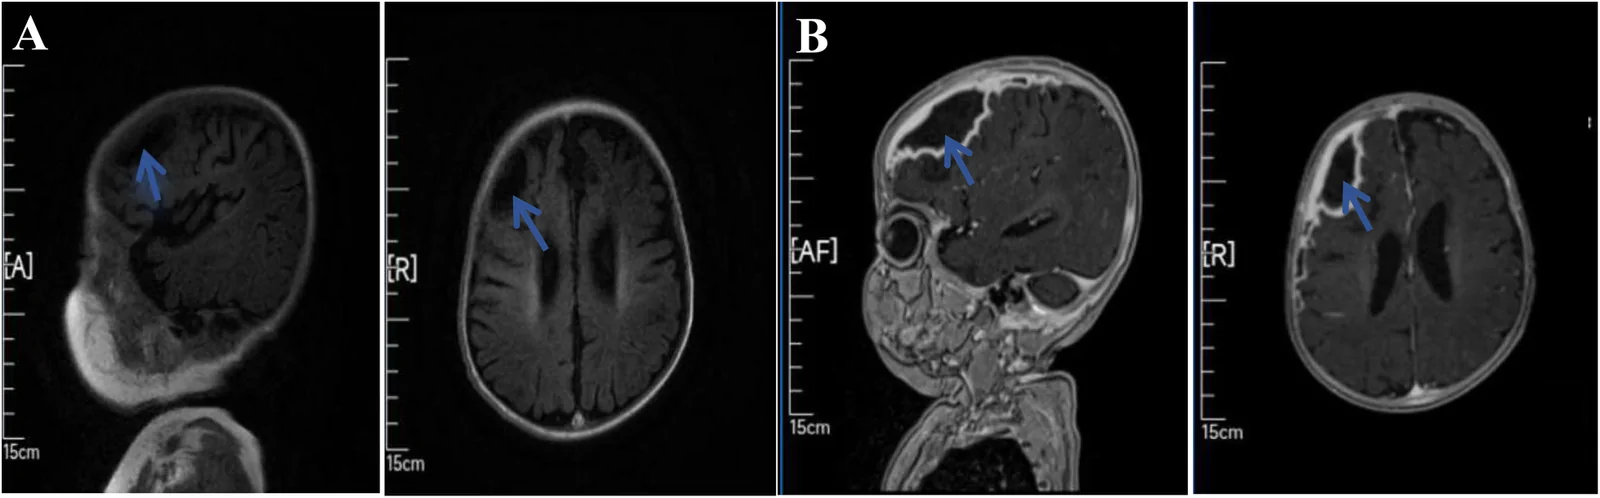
Pre-operative cerebral MRI findings in the preterm infant with *E. coli* meningitis complicated by intracranial abscess. (A) Pre-operative T1-weighted imaging revealed suspicion of right frontal epidural and right frontotemporal subdural abscess, with thickening of the cerebral falx. (B) Contrast-enhanced T1-weighted imaging acquired one week after panel (A) demonstrated progressive enlargement of the right frontotemporal epidural and subdural abscesses, with adjacent brain parenchyma compression and edema, thickening and enhancement of the dura mater, leptomeninges, and falx cerebri.
